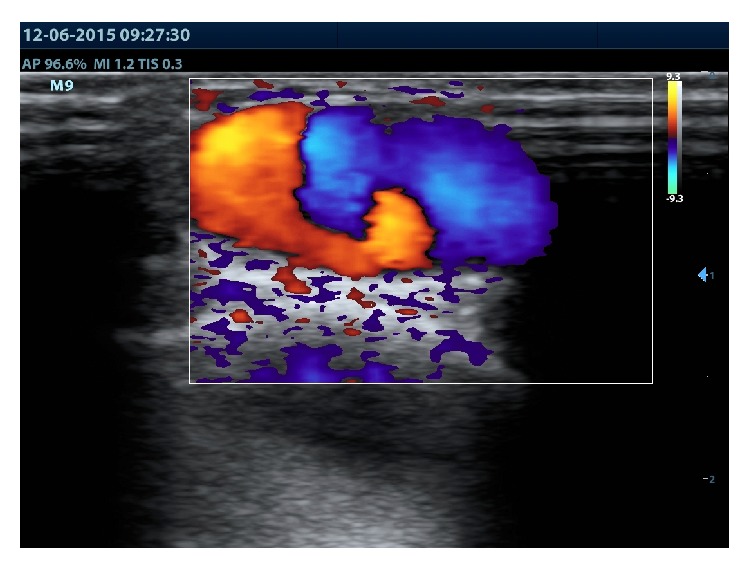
Turbulent flow inside the sac of aneurysm (“yin-yang” sign) detected by duplex ultrasound during the compression of venous foot pump.
